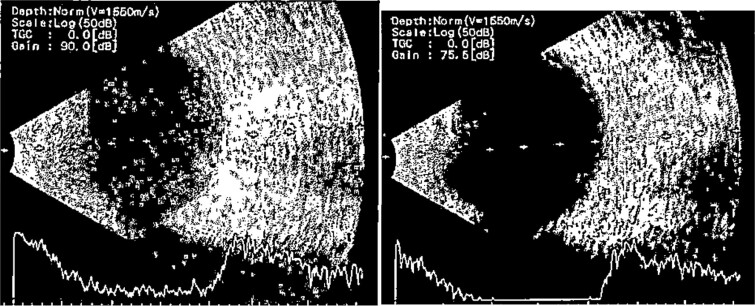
B-scan ultrasound demonstrating mild to moderate vitritis in endophthalmitis case for patient 3.
